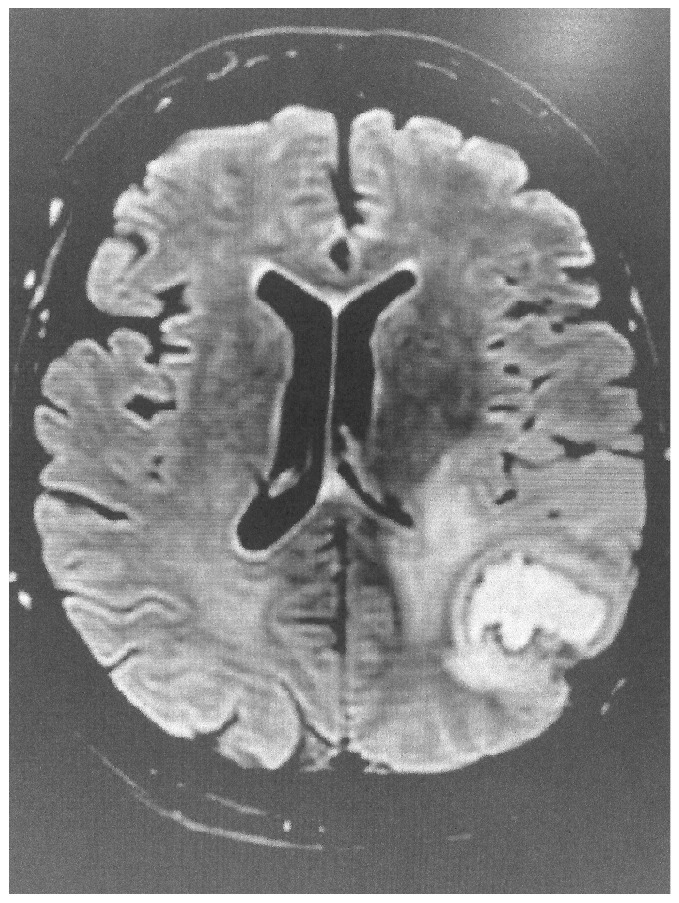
Brain FLAIR image showing evidence of stroke.
